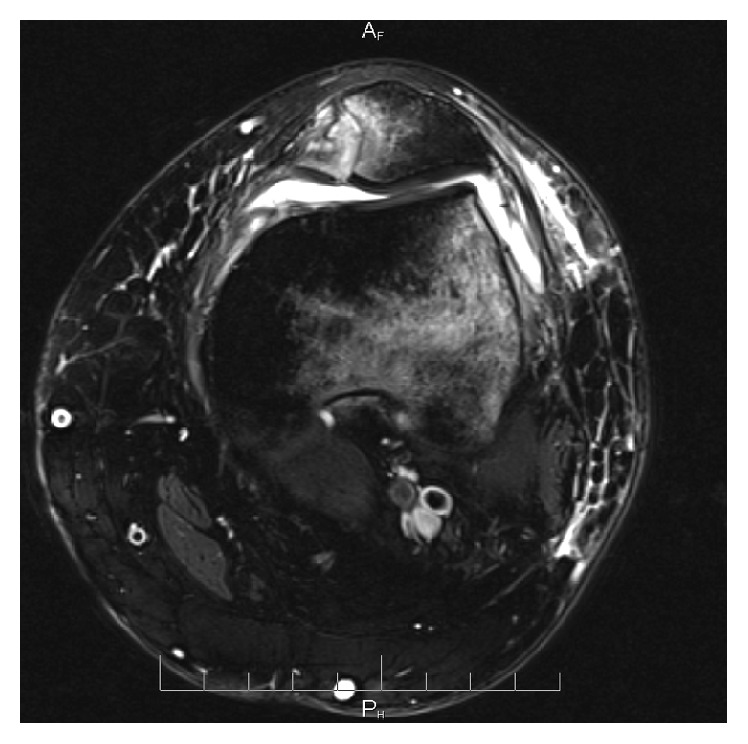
Axial cut of a T2 weighted MRI obtained at follow-up which demonstrates an acute fracture of the medial facet of the patella and characteristic edema of the lateral femoral condyle.
